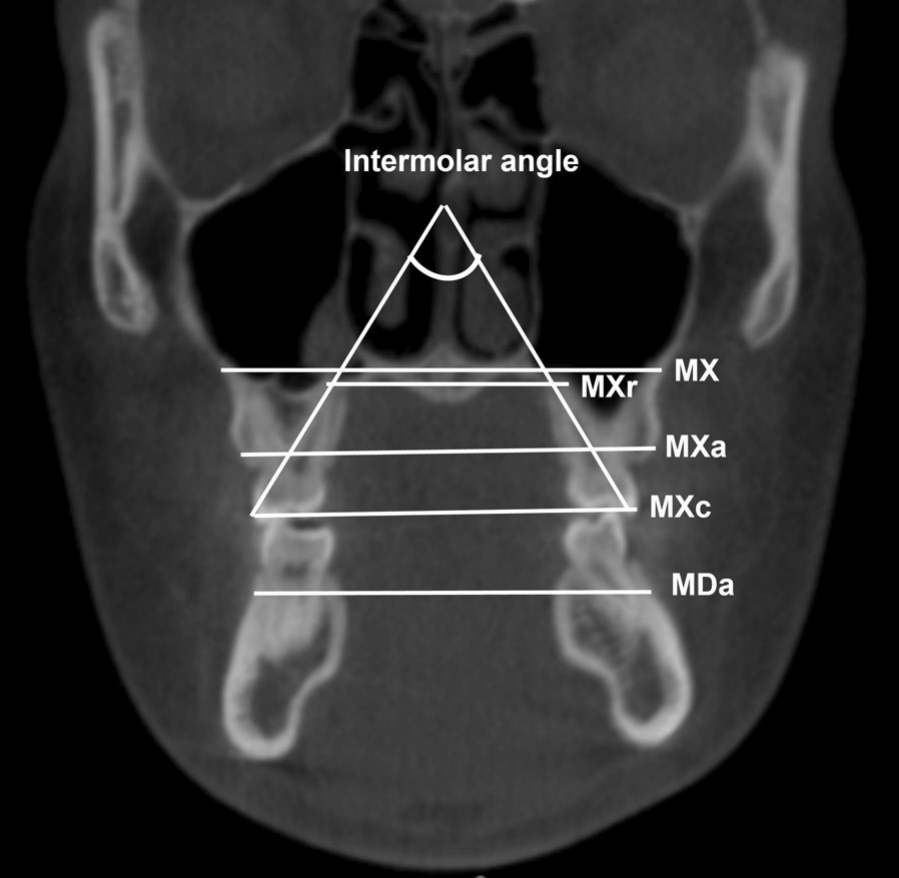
Cone beam computed tomography image in coronal view showing the linear measurements: maxillary base width (MX), maxillary alveolar width (MXa), maxillary root width (MXr), maxillary tooth cusp width (MXc), mandibular alveolar width (MDa) and angular measurement: intermolar angle (IA)
